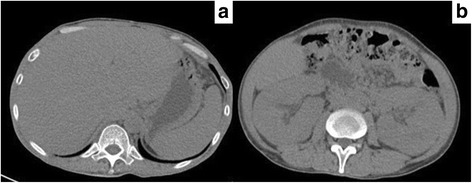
The enlargement of liver and bilateral kidneys (a, b) detected by using computed tomography
